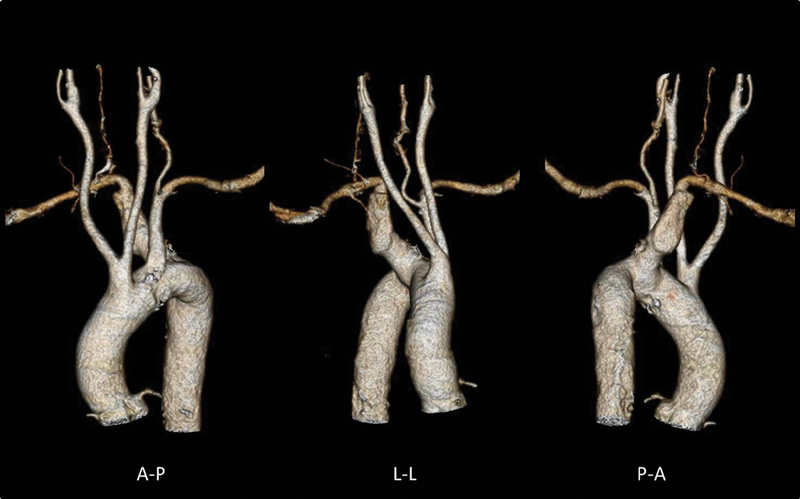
Preoperative angio–computed tomography three-dimensional reconstruction. A-P, anterior-posterior; L-L, lateral-lateral; P-A, posterior-anterior.
